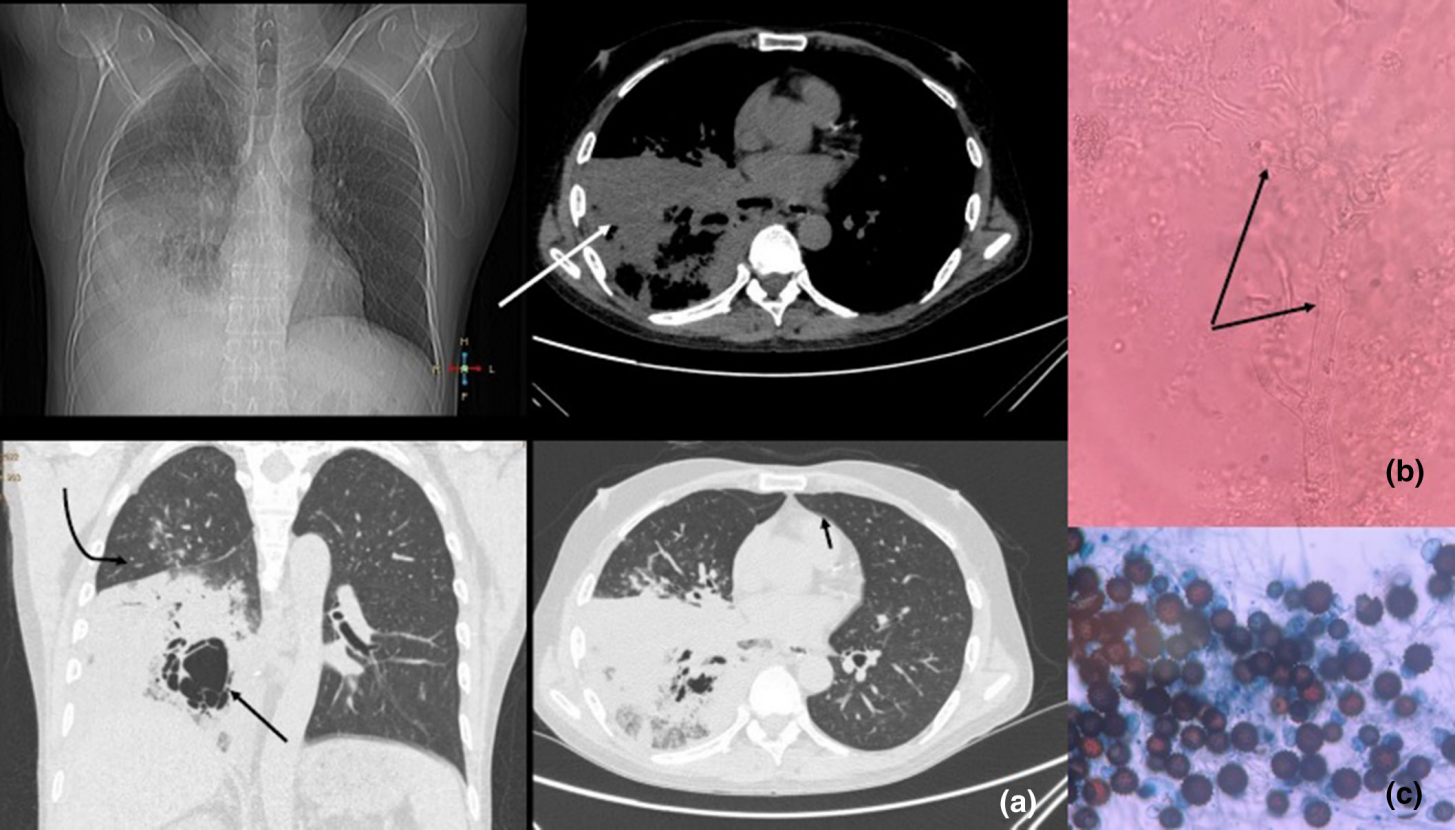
(a) Chest radiograph (first spot) showing non-homogenous opacification of right mid-lower zone with air bronchogram and obscured right costophrenic angle and right hemidiaphragm s/o Lobar pneumonia. HRCT of the chest with mediastinal and lung windows (second to fourth spots) showing dense opacification of right lower lobe (white arrow) limited by horizontal fissure s/o lobar pneumonia with multiple irregular cavities within it (long black arrow). Patchy area of consolidation seen in right upper lobe on coronal image (curved black arrow). (b) KOH mount of bronchoalveolar lavage, with arrows showing broad non-septate hyphae, which gives presumptive evidence for mucormycosis. (c) Lactophenol cotton blue (LPCB) mount showing *R. homothallicus* with characteristic golden brown, globose zygospores with stellate spines and suspensor cells (100×).
